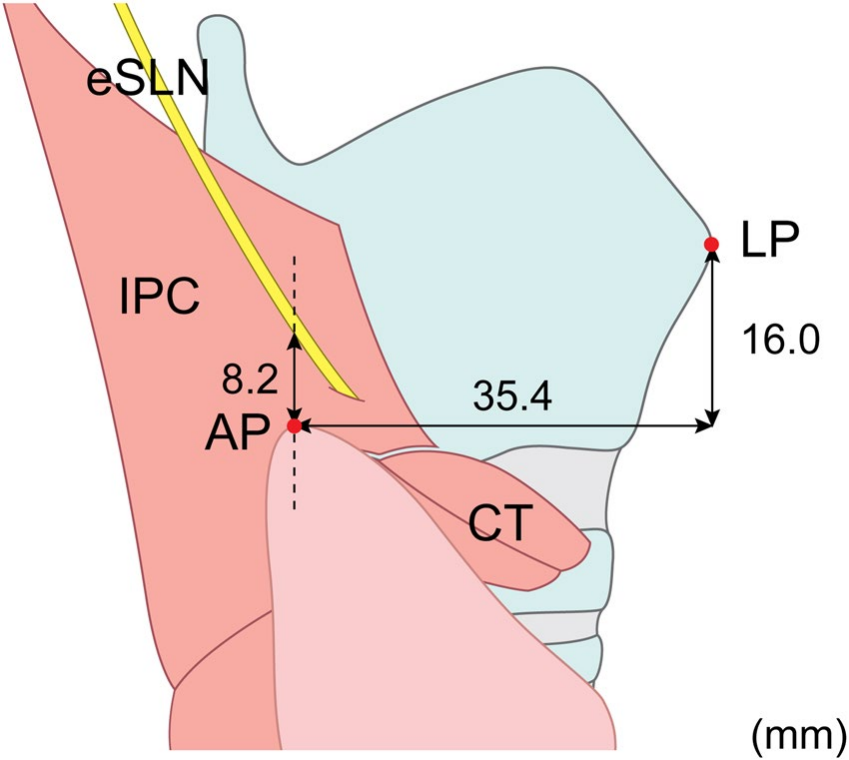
The position of the apical pole (AP) of the thyroid gland and the external branch of the superior laryngeal nerve (eSLN) into the laryngeal muscle. LP, laryngeal prominence; IPC, inferior pharyngeal constrictor; CT, cricothyroid.
